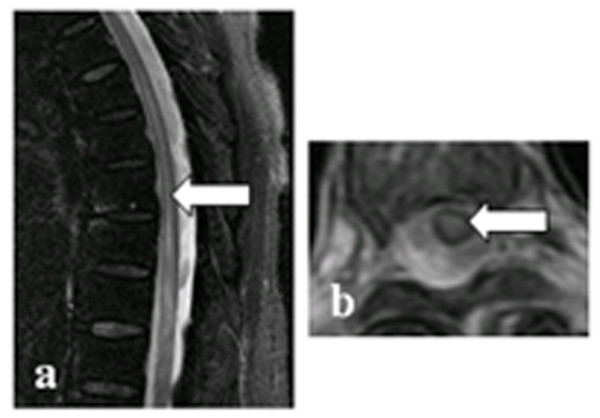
Spinal cord lesion in an NMO patient. The lesions are shown by arrows. a: T2-weighted image showing a linear-shape lesion; b: axial T2-weighted image showing a cervical lesion predominantly involving the central gray matter in the spinal cord.
